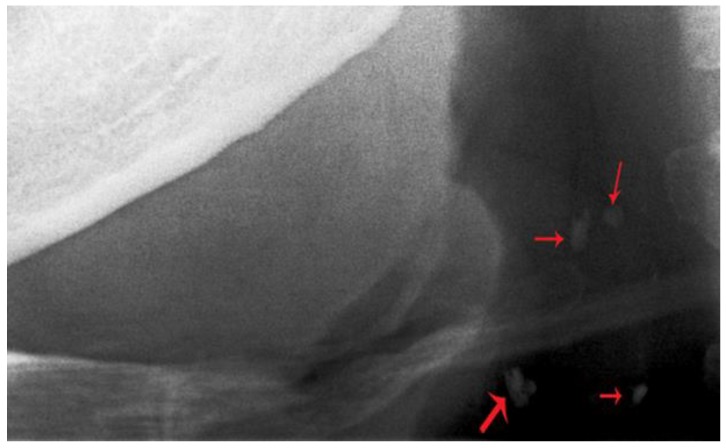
Close-up view of a panoramic radiograph showing the radio-opacities in the carotid vasculature. Red arrows indicate carotid artery calcifications.
